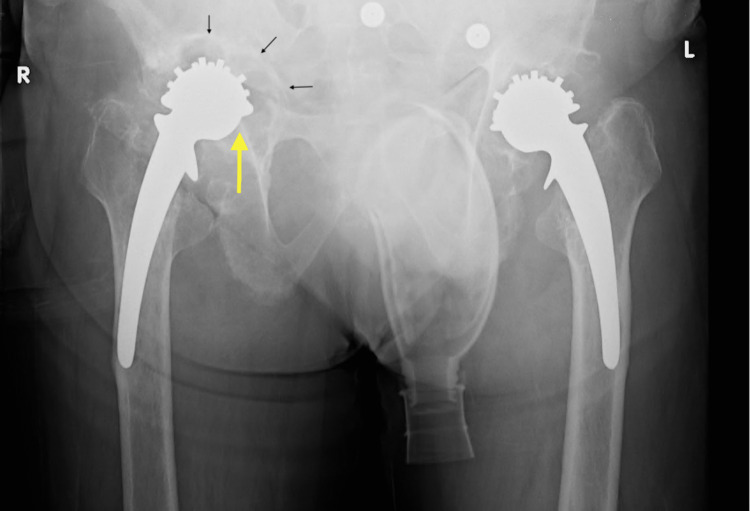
Radiographs on presentation showing retro-acetabular osteolysis (black arrows) with disruption of Kohler's line (yellow arrow) and ischial lysis on the right side with similar but less remarkable findings on the left
